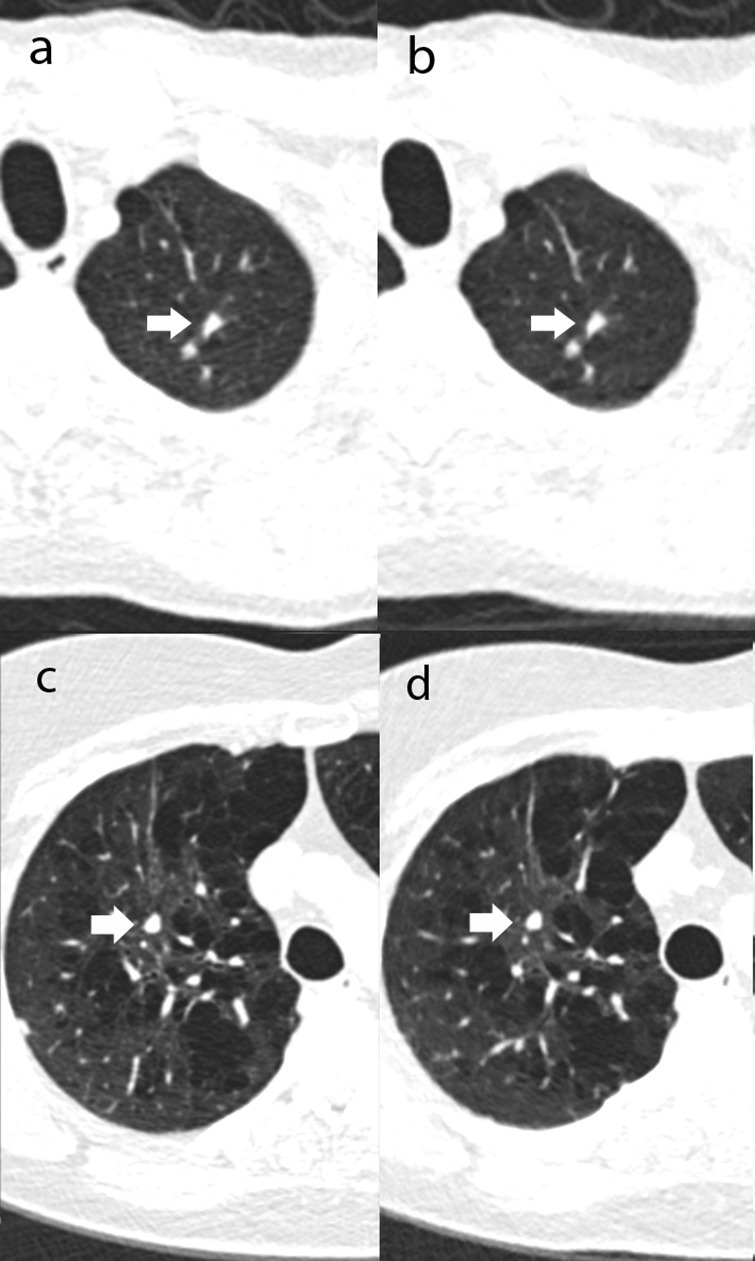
Transverse thorax CT images with nodules missed on ULDCT but found on RDCT from two patients. (a) and (c) are both RDCT. (b) and (d) are both ULDCT, assumed to be missed due to close proximity with apical lung fibrosis, and with bronchovascular bundle, respectively. RDCT, response-dose CT; ULDCT, ultralow-dose CT.
